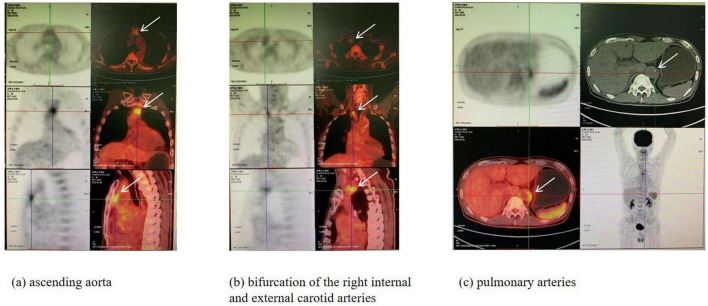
PET/CT of the patient (2022.02.04). Multiple nodular and strip-shaped hypermetabolic lesions were observed at the bifurcation of the right internal and external carotid arteries, as well as in the ascending aorta, pulmonary arteries, and descending aorta. There were also strip-shaped and nodular hypermetabolic lesions in the pericardium. (a) The arrows indicate hypermetabolic foci in the ascending aorta. (b) The arrows point to hypermetabolic foci at the bifurcation of the right internal and external carotid arteries. (c) The arrows highlight hypermetabolic foci in the pulmonary arteries.
